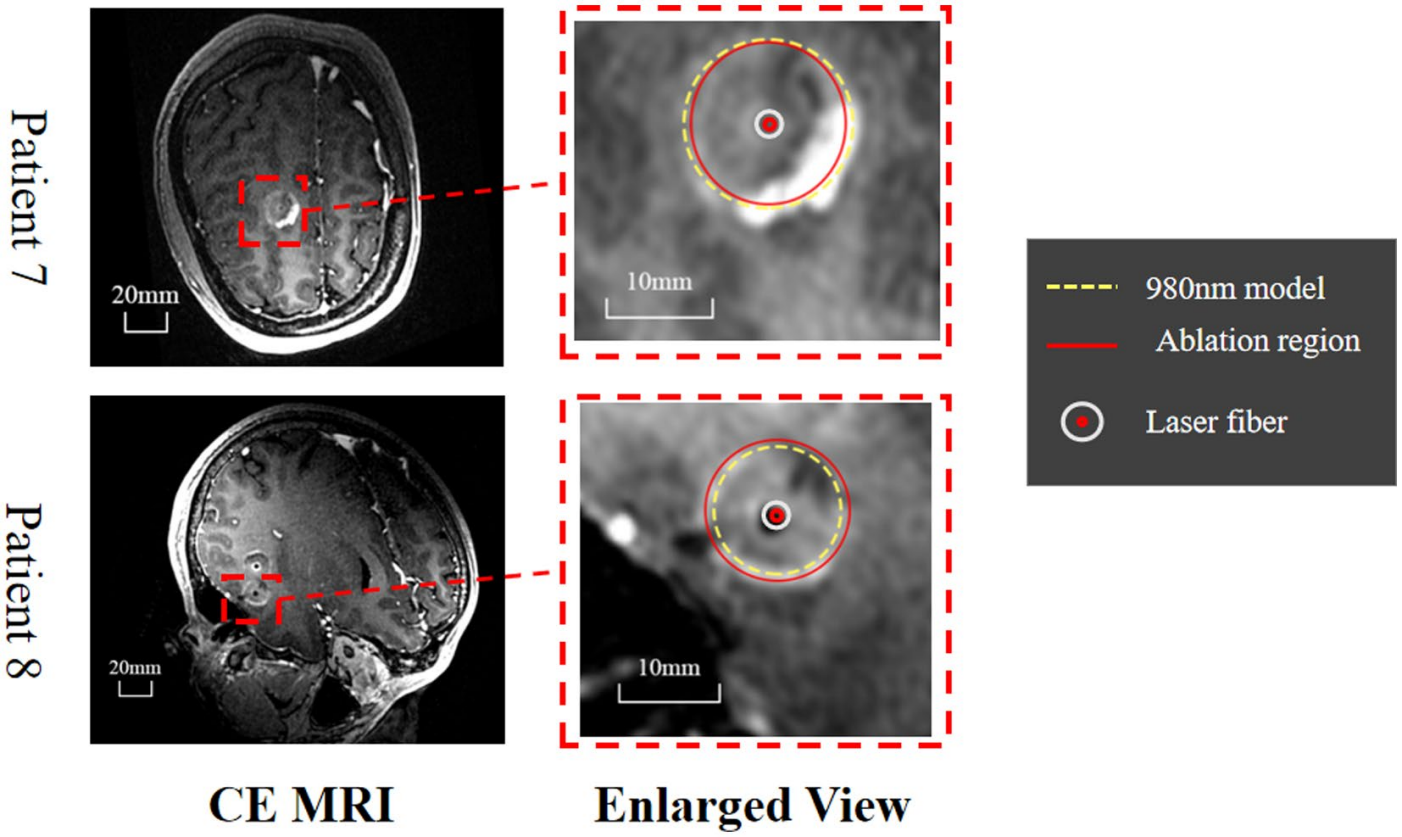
Evaluation of the predicted ablation area on two epileptic patients. The CE MRI acquired immediately after surgery was used to determine the real ablation boundary (red, curve). The ablation boundary predicted by our proposed model (980 nm, yellow dashed curve) showed good agreement with the CE MRI measurements.
